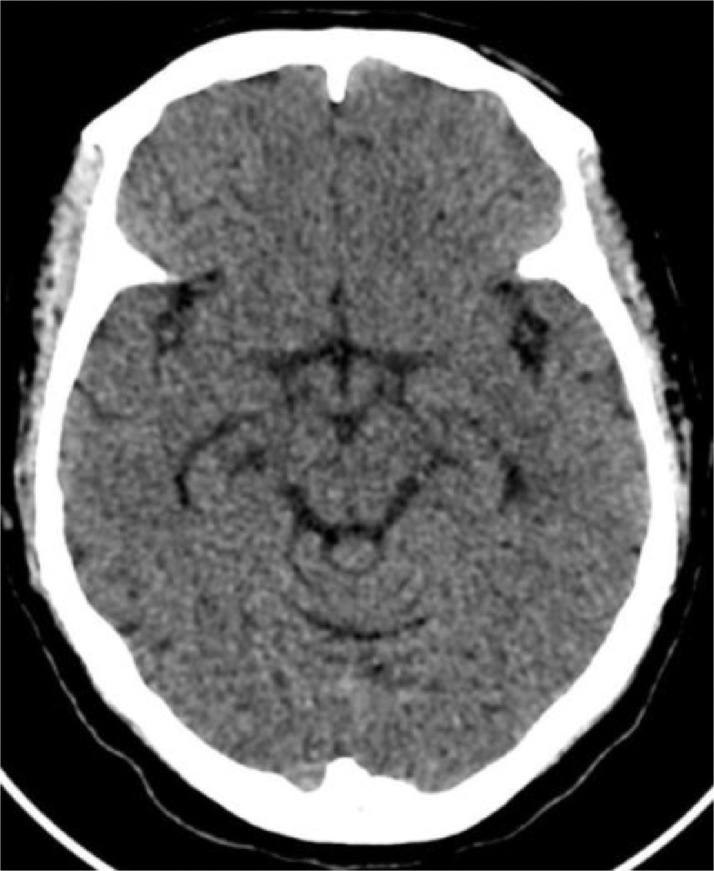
Axial CT image of the brain without contrast was unremarkable.
